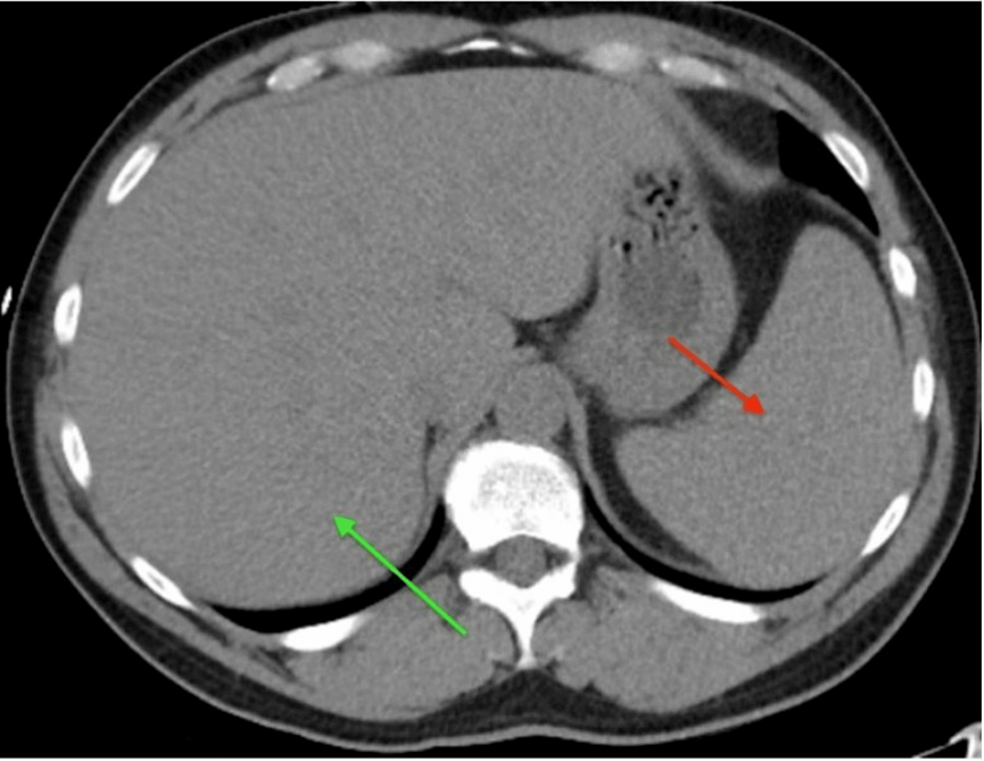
CT abdomen showing splenomegaly (red arrow) and liver with mild hepatomegaly (green arrow)
